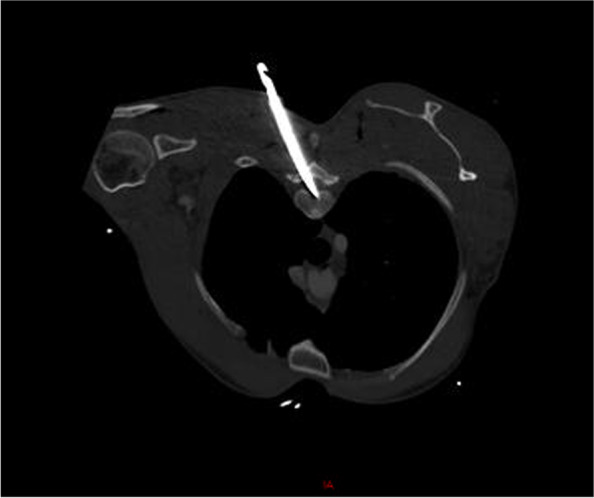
Axial CT scan of the thorax demonstrating foreign body(knife) in the spinal canal in an oblique position reaching the anterior aspect of the spinal canal
